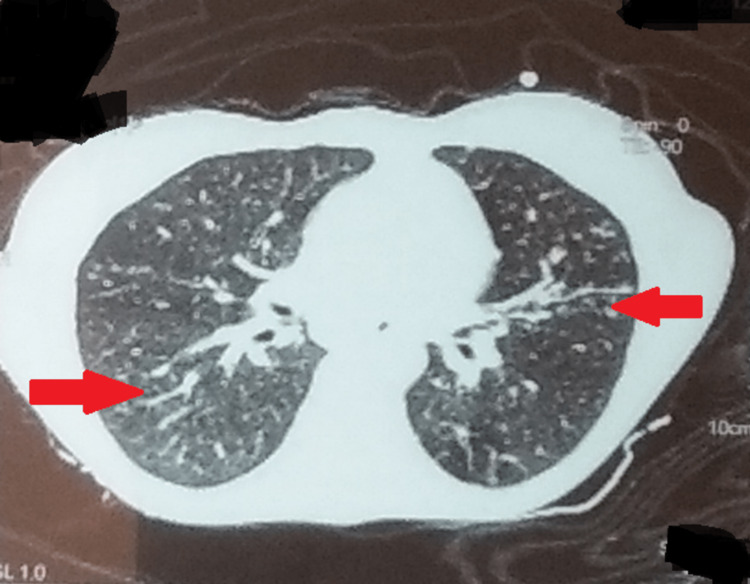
CECT chest showing bilateral involvement of the lungs CECT: contrast-enhanced computed tomography
